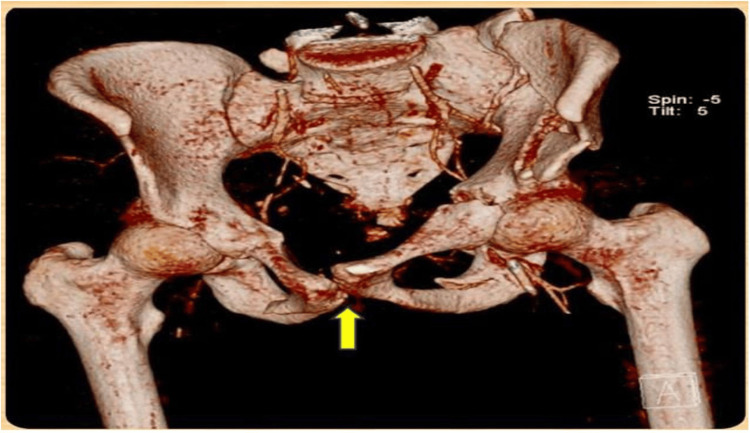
3D CT scan of PF with displacement. The yellow arrow indicates the fracture site PF: pelvic fracture
